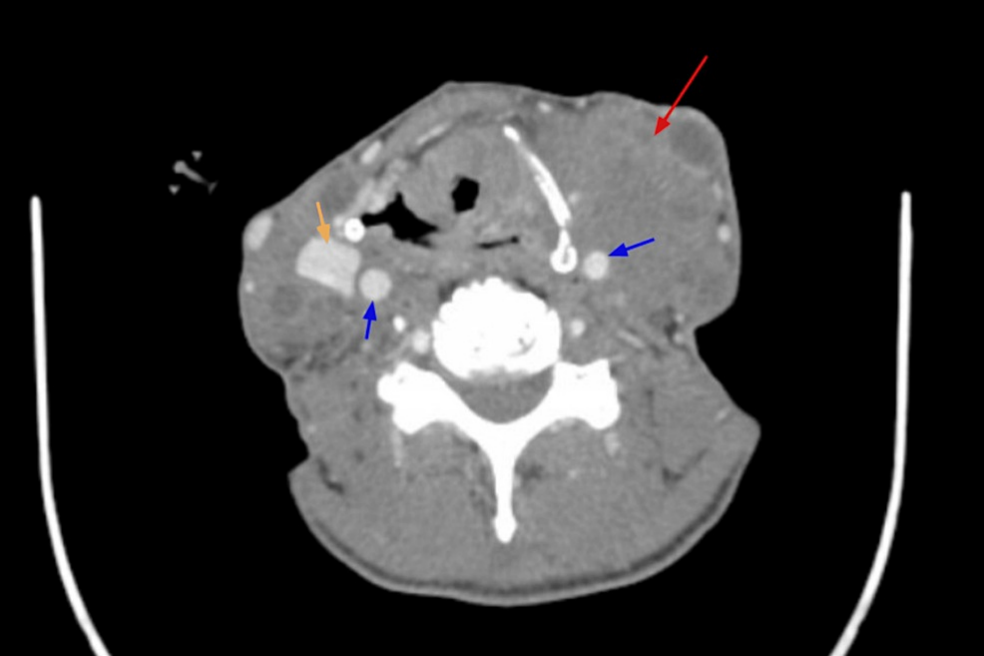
CT scan of the neck with contrast (axial view): large mass (red arrow) near the carotid artery (blue arrows). The internal jugular vein (orange arrow) is seen on the right side.
